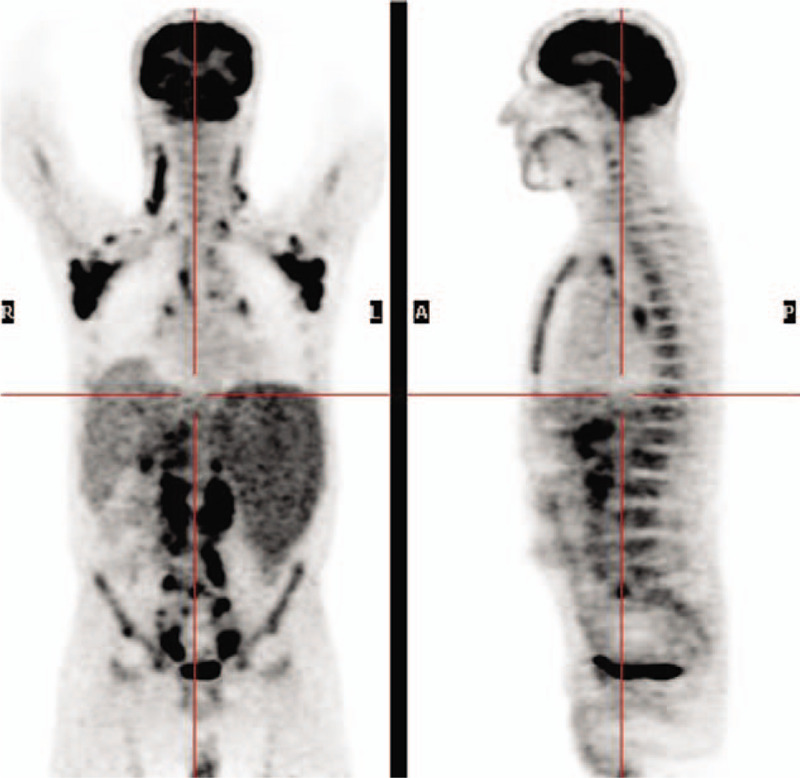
Positron emission tomography/computed tomography scan at diagnosis showed accumulation of fluorodeoxyglucose in several deep and superficial lymph node locations (SUV max range 2–7.7).
